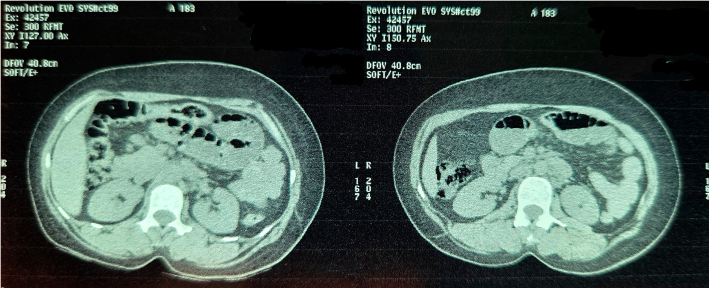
Non Contrast Computed Tomography of Abdomen and Pelvis (Transverse Section Zoomed In).
